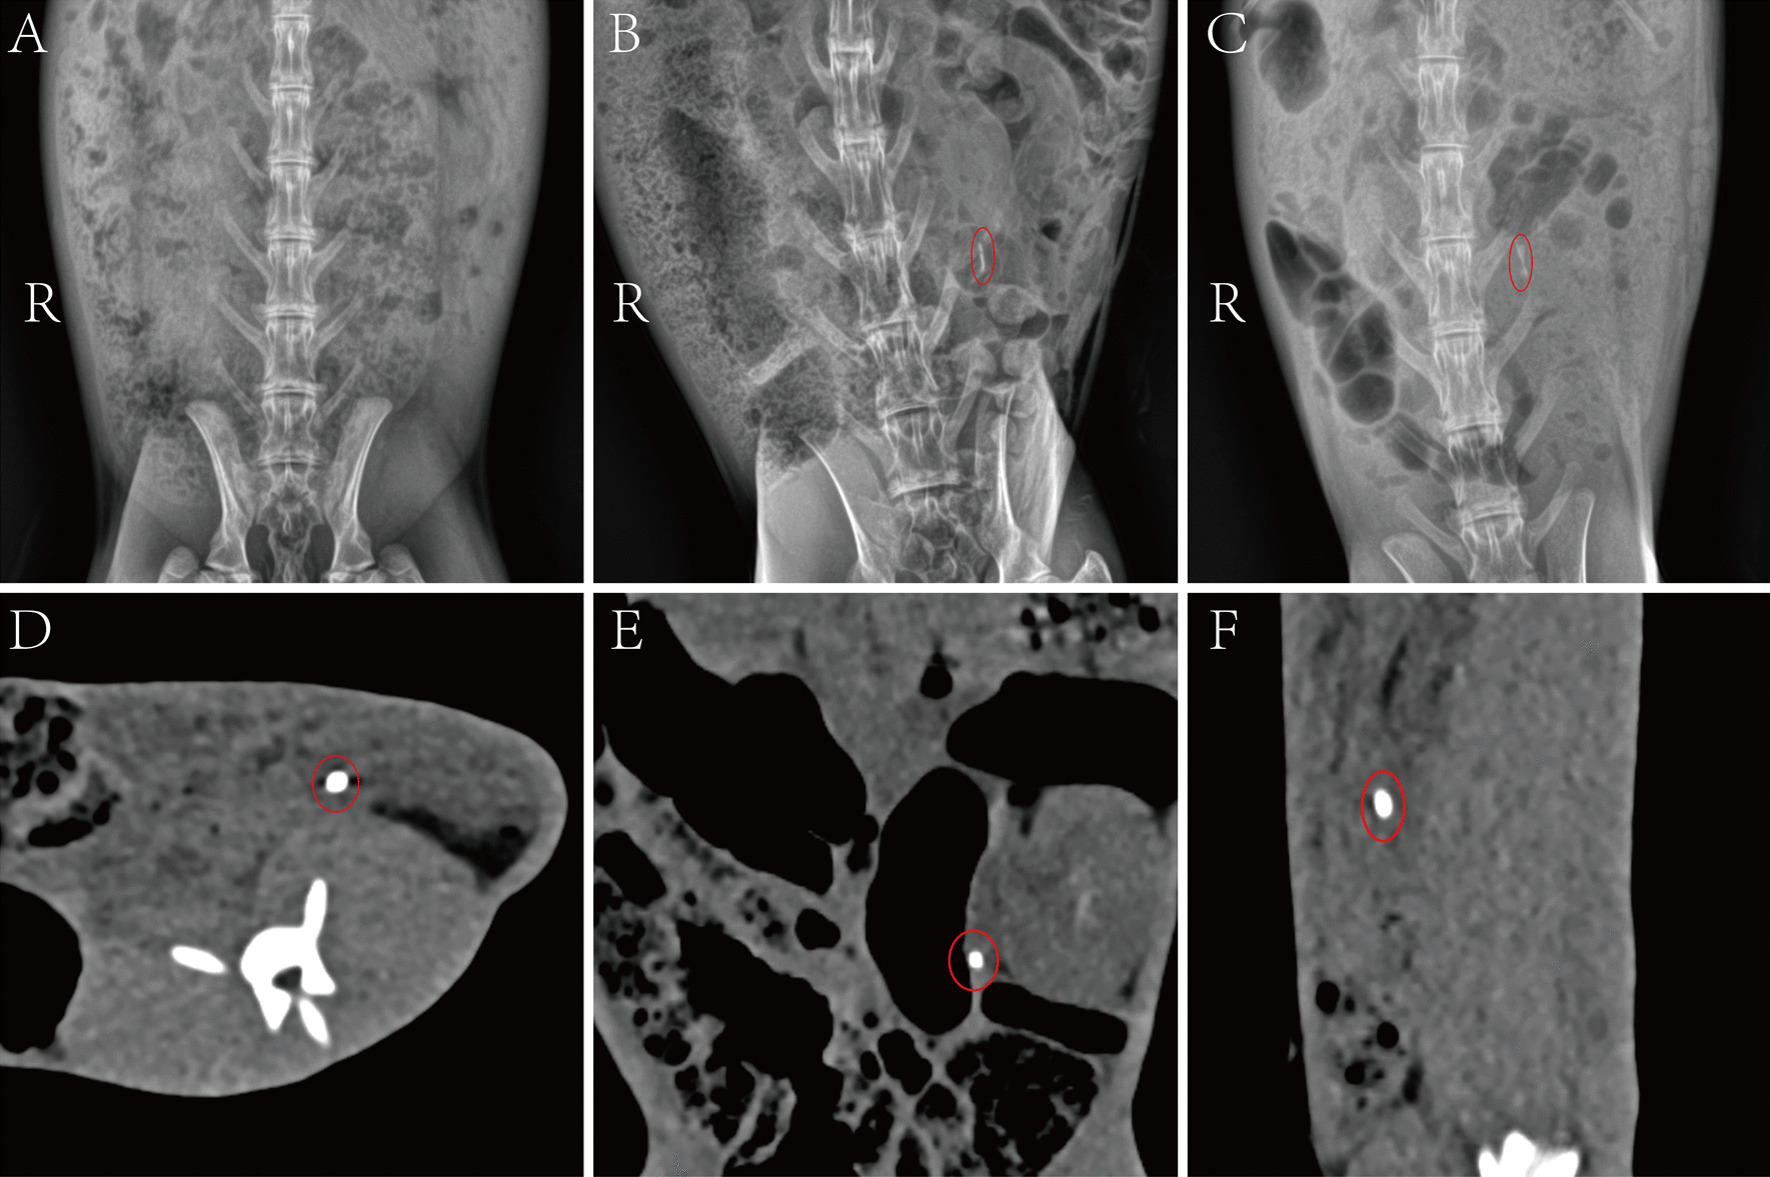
Ureteral stones were assessed by X-ray and CT imaging. A The preoperative x-ray. B The end of the surgery X-ray. C The 7th postoperative day X-ray. The 7th day postoperatively CT examination of cross-sectional (D), Coronal (E) and sagittal scans (F). The high-density shadows of stones were marked by red circles
